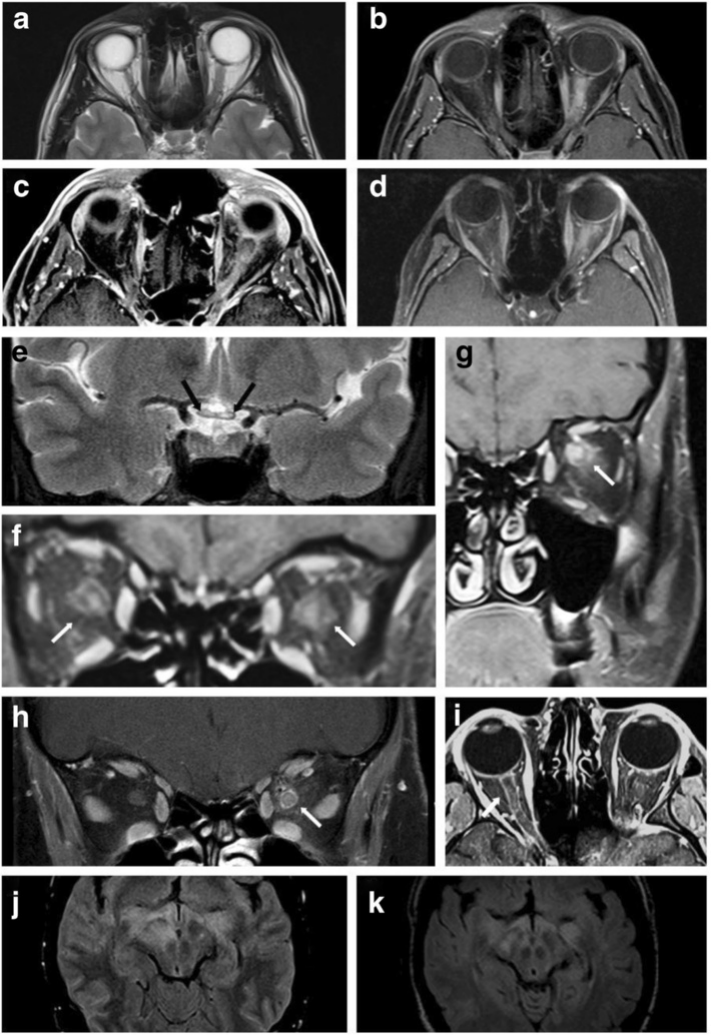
Examples of optic nerve lesions detected by MRI. a, b T2-weighted (a) and T1-weighted (B, with Gd) MRI reveals swelling and Gd enhancement of the left optic nerve. c, d (fat-suppressed): Longitudinal extensive Gd enhancement of the optic nerve (see cases 9 and 12 in part 3 [31] for details). e Longitudinally extensive bilateral optic neuritis extending from the chiasm (E, *black arrows*) into the orbits, affecting the left more than the right optic nerve. f-h Coronal T1-weighted MRIs display marked contrast enhancement of the intraorbital optic nerve as well as concurrent enhancement of the perioptic nerve sheath, partly extending in the surrounding orbital fat, in patients with acute ON (cases 11, 29 and 19). I: Axial T1-weighted MRI shows Gd enhancement along the right optic nerve in another patient (see case 13 in part 3 of this article series [31]). j, k Axial FLAIR imaging demonstrates bilateral lesions in the optic tract (see case 8 in part 3 [31] for details) (j MRI at attack onset; k follow-up MRI 1 month later)
